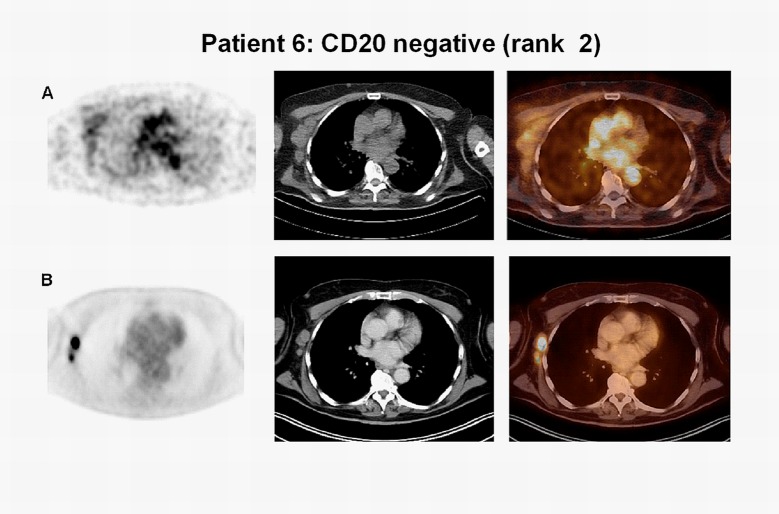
Tumor uptake on ^89^Zr-rituximab-PET, discordant with CD20 expression in biopsy. Axial images, from left to right attenuation corrected PET, low dose CT and fused PET/CT image of patient 6. a) ^89^Zr-rituximab-PET shows tumor uptake discordant with a CD20-negative biopsy (axillar lymph node). b) Corresponding tumor location on ^18^F-FDG-PET.
